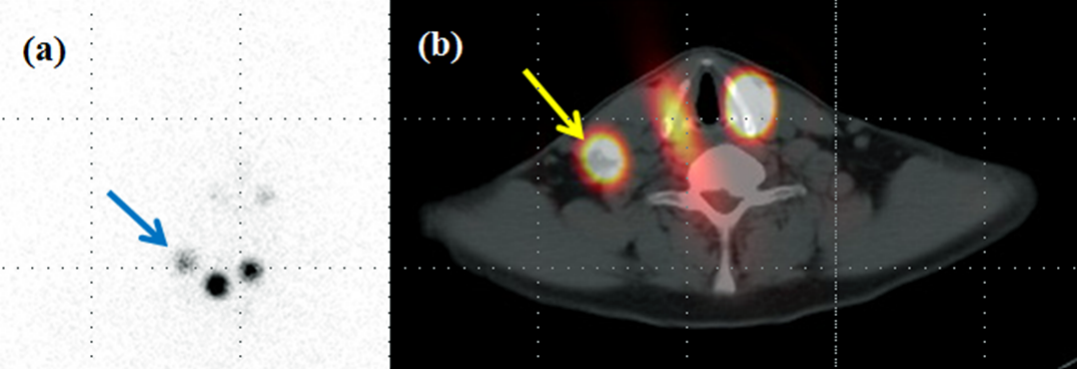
Postablation I-131 whole body planar scan and SPECT/CT of a patient with papillary thyroid cancer. Preablation TSH-stimulated serum thyroglobulin level was 4.7 ng/ml. A focal iodine uptake was seen in the right neck on postablation I-131 planar scintigraphy (a). The SPECT/CT image also revealed RAI uptake on a small LN in the right upper neck (b).
